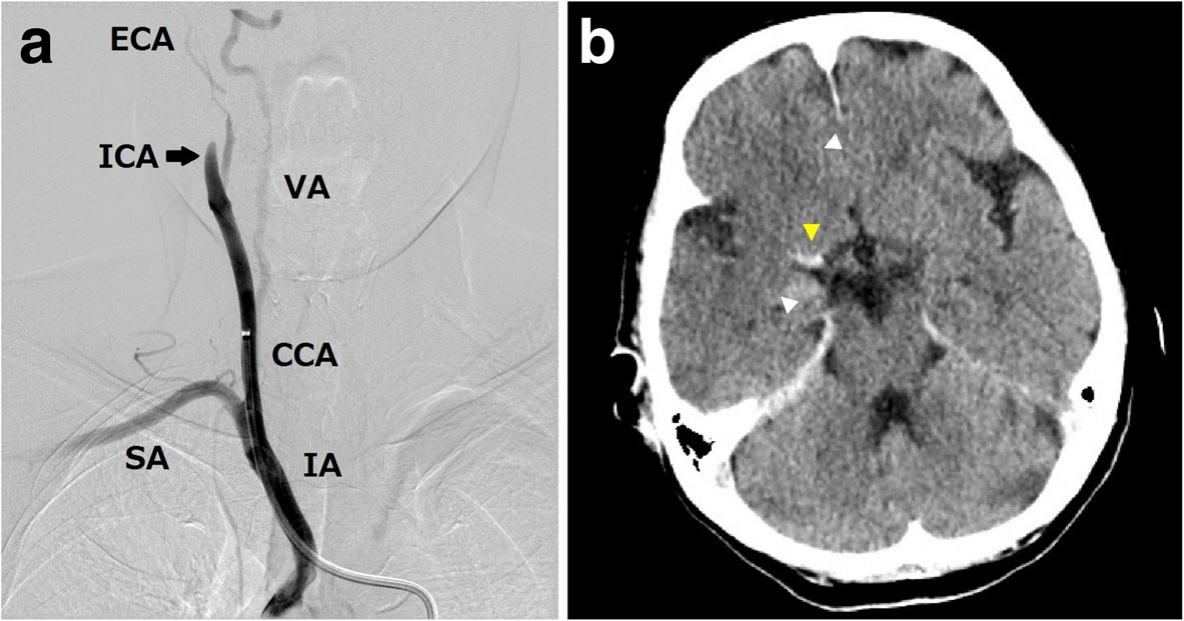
Thrombosis of the right internal carotid artery (ICA) in case 2. a Angiogram of the right cerebral circulation showing occlusion of the right ICA (arrow). b Head computed tomography after thrombectomy revealing cleared ischemic lesion (white arrowheads) and a thrombus at the right middle cerebral artery (yellow arrowhead). IA, innominate artery; SA, subclavian artery; CCA, common carotid artery; VA, vertebral artery; ICA, internal carotid artery; ECA, external carotid artery
